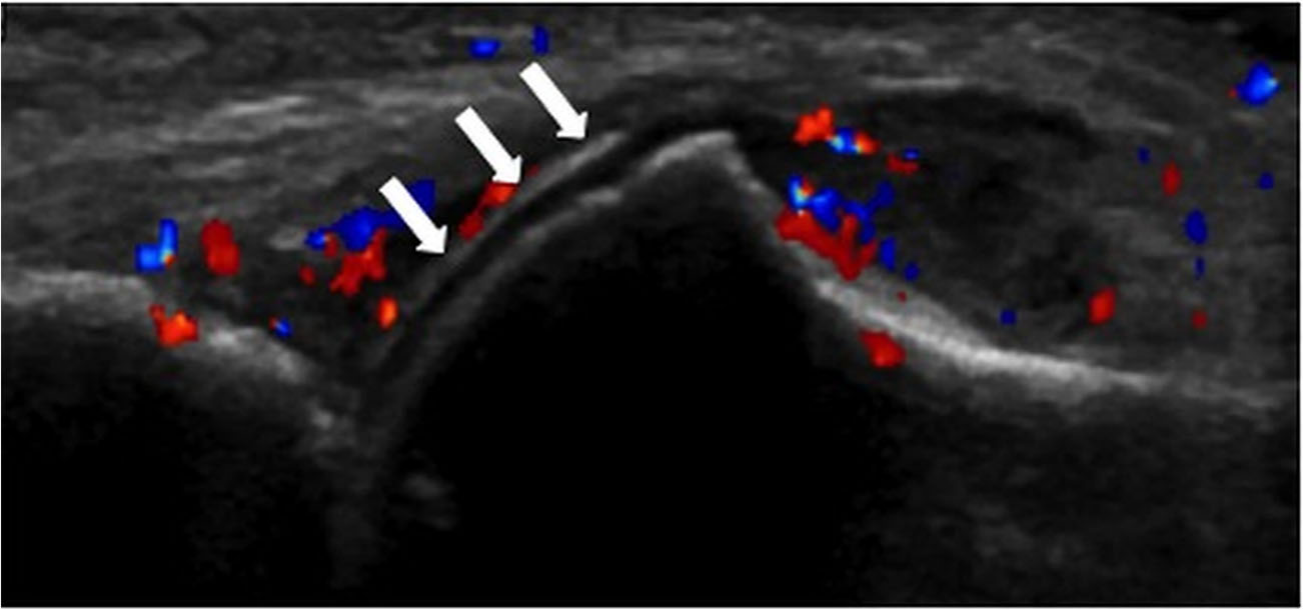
Right hand of a 77-year-old male patient. Longitudinal ultrasound (US) scan of metacarpophalangeal (MCP) joint showing double contour sign (arrows). Neither tophi nor aggregates can be seen. Dual-energy CT (DECT) of the same joint (not shown) was negative
